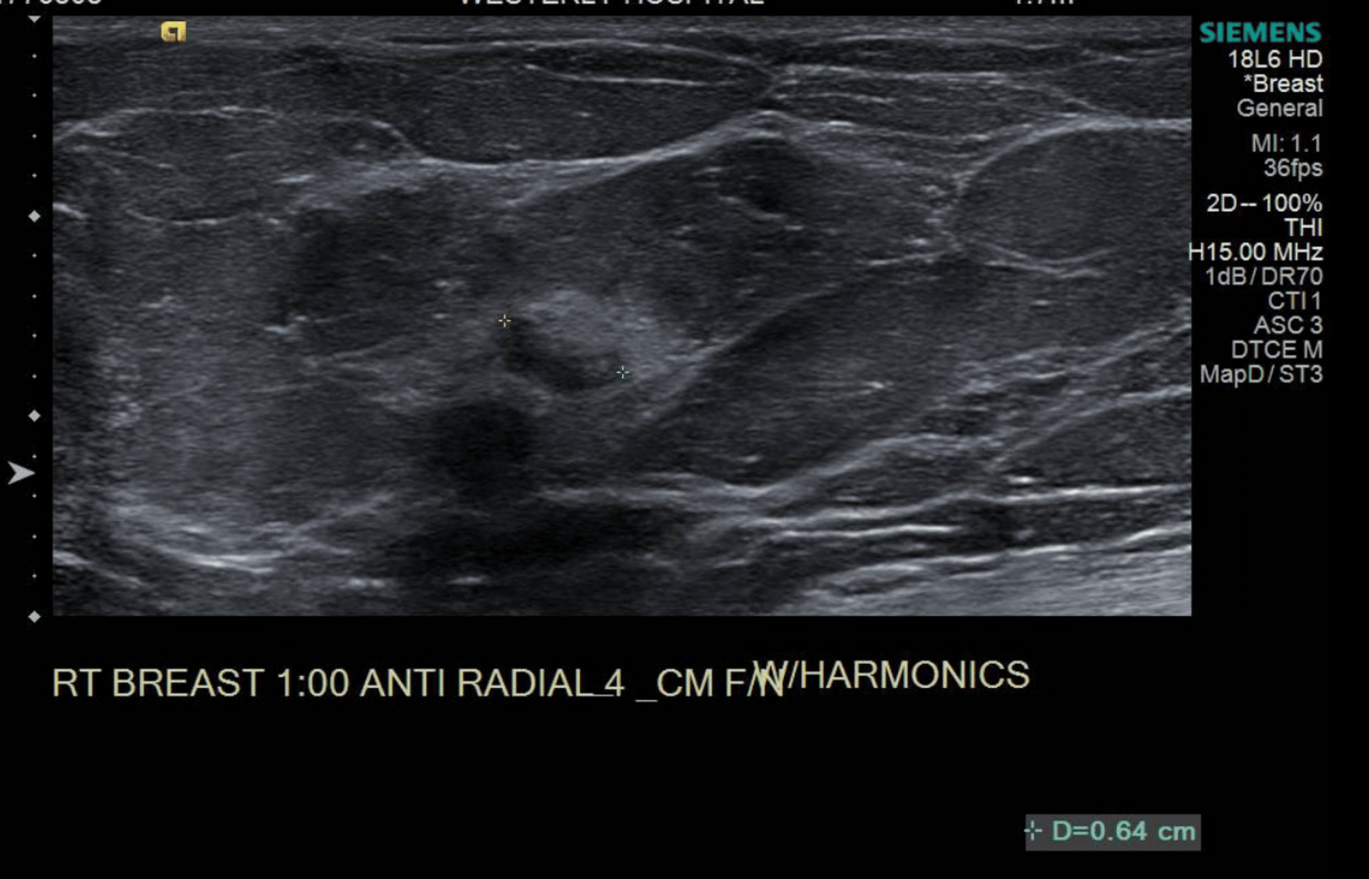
Ultrasound of the right breast for the one o'clock lesion. This ultrasound further delineates the one o'clock lesion in the right breast, which was biopsy-proven to be marginal zone lymphoma. The crosshairs give an approximate dimension.
